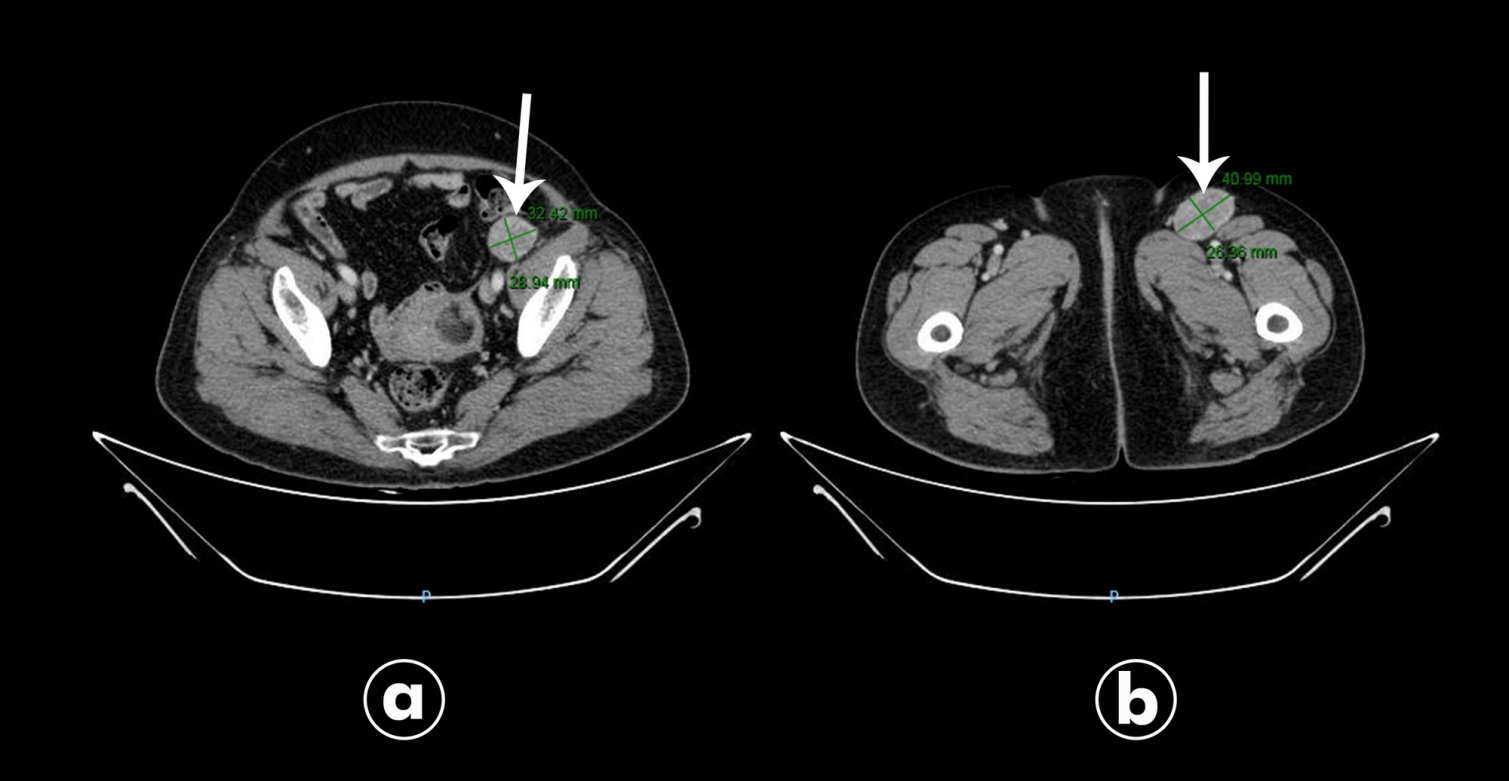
CT of the pelvis with heterogenous lymph nodes enlargement: (a) in the left external iliac chain, and (b) in the left inguinal region.
